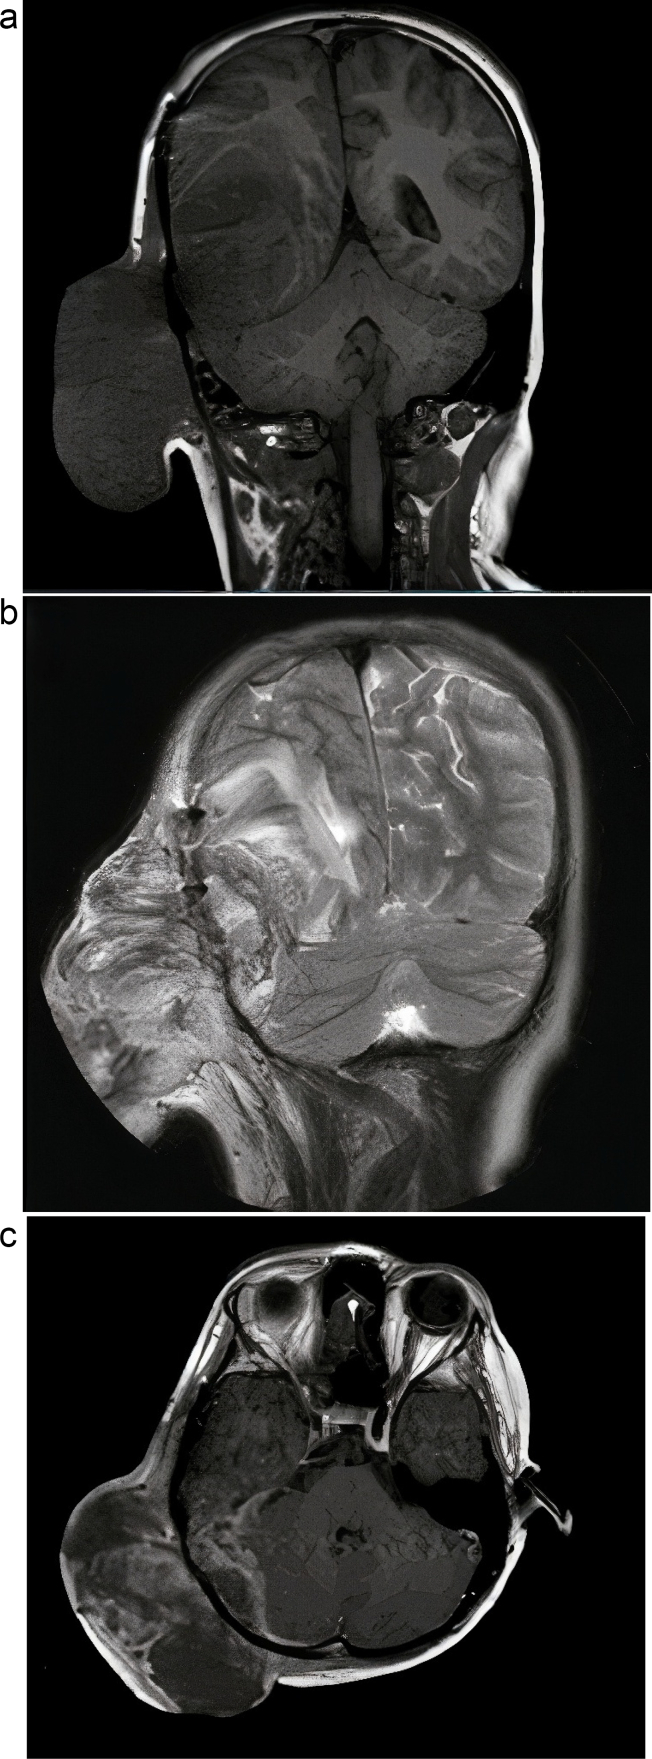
Magnetic resonance imaging following tumor relapse. (a) T1 (coronal view). (b) T2 (coronal view). (c) T1 (axial view) + gadolinium (contrast) injection.
